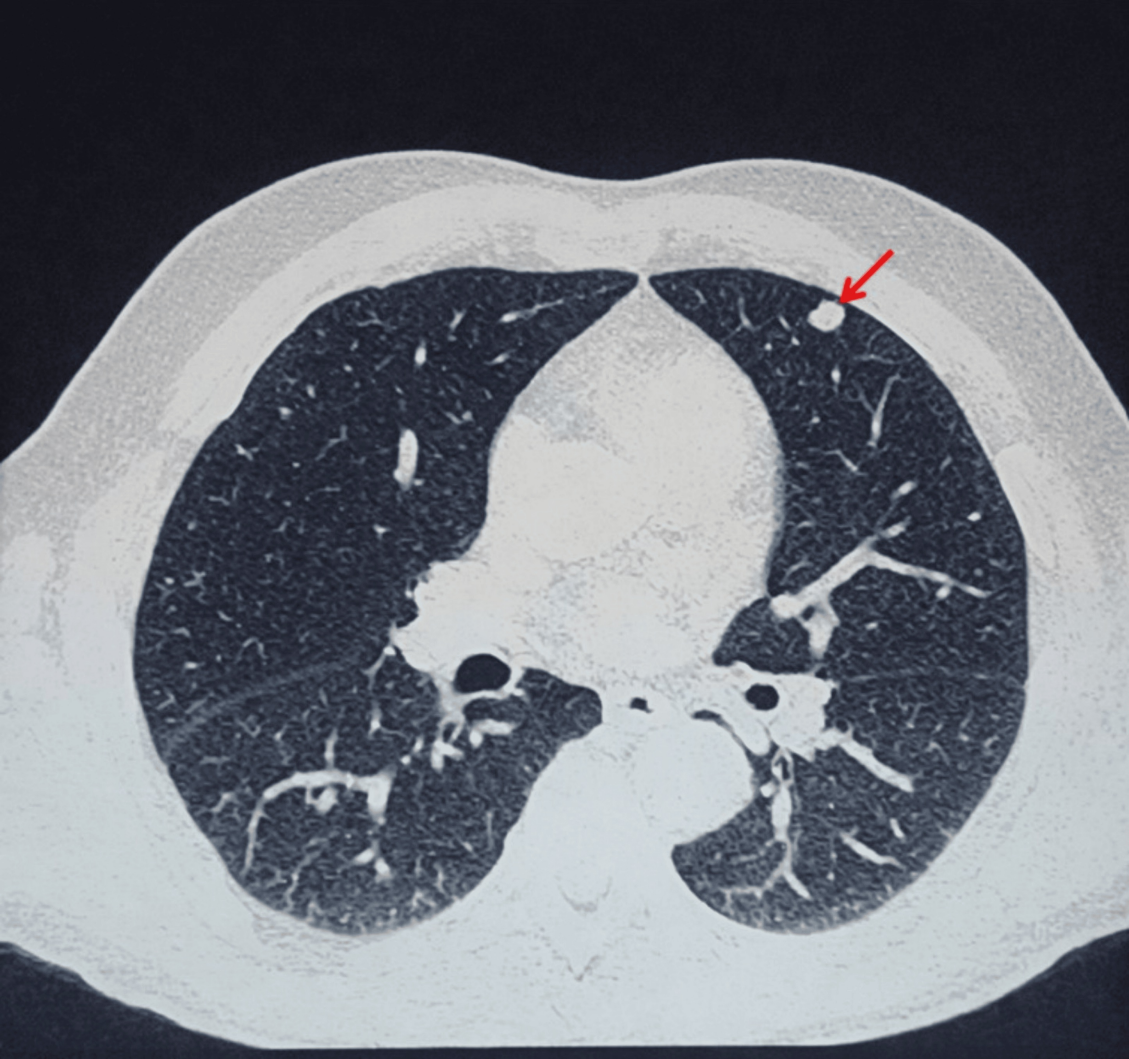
An axial chest CT section demonstrating a well-circumscribed bilobed pulmonary nodule located in the lingula (red arrow), measuring 0.8 x 0.7 cm, in a 62-year-old male
